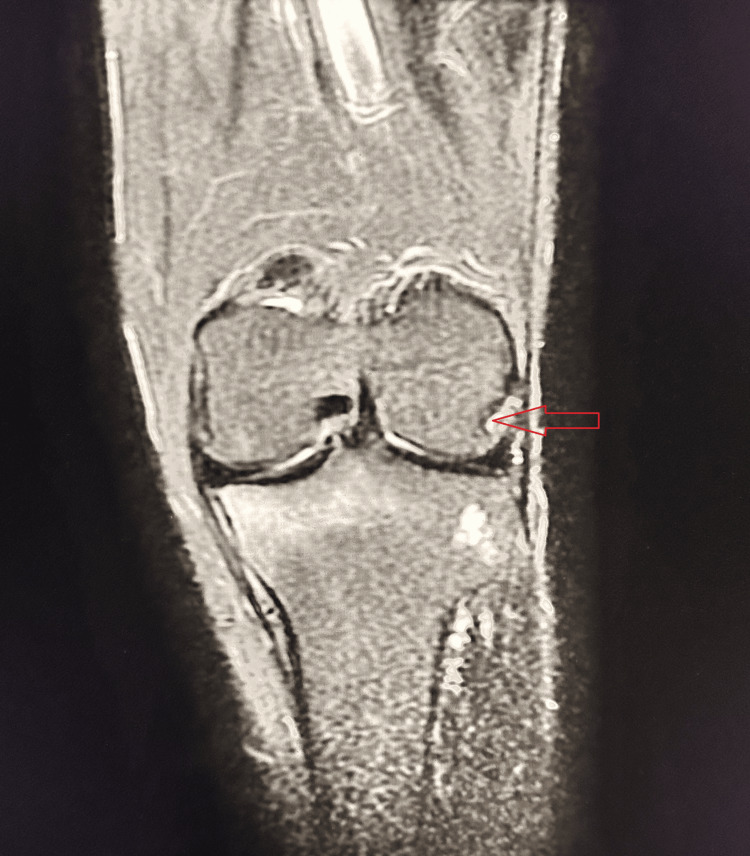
Grade II osteoarthritis of the knee joint Coronal T2-weighted MRI demonstrates reduced joint space in the medial and lateral compartments with marginal osteophyte formation along the femoral condyles and tibial plateau, consistent with KL Grade II osteoarthritis (red arrow). KL, Kellgren-Lawrence
